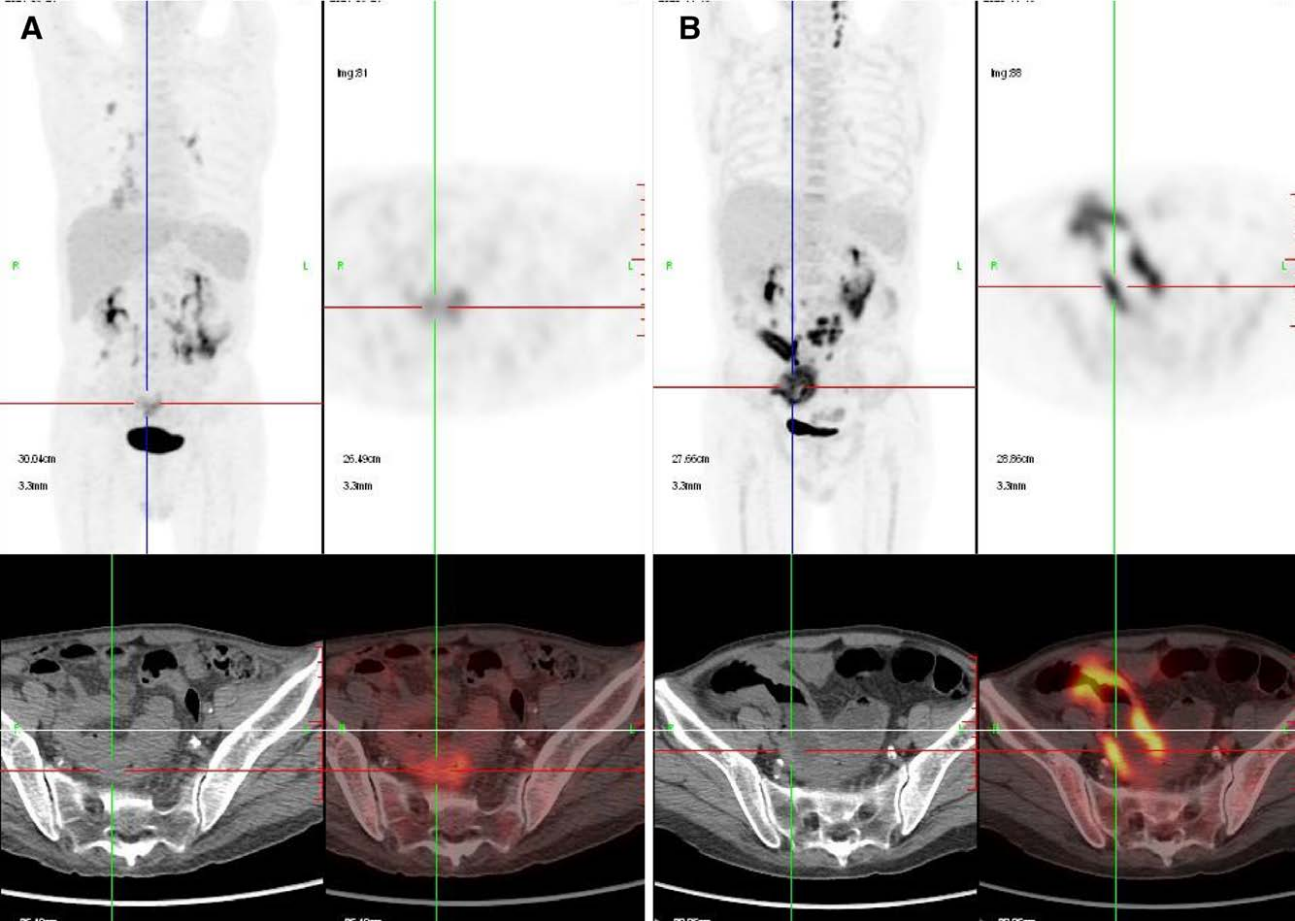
PET/CT images show decreased SUV at retroperitoneal and mesenteric lymph nodes in May 2021 (A) compared to the images in November 2020 (B) after daratumumab-based treatment. The SUV max of retroperitoneal and mesenteric lymph nodes was 16.6 (3.0 cm × 1.7 cm) and decrease to 6.8 (1.8 cm × 1.1 cm). Also, the tumor infiltration in the oropharynx subsided. Partial remission was achieved after 3 cycles of daratumumab-based therapy. PET/CT = positron emission tomography/computed tomography, SUV = standardized uptake value.
